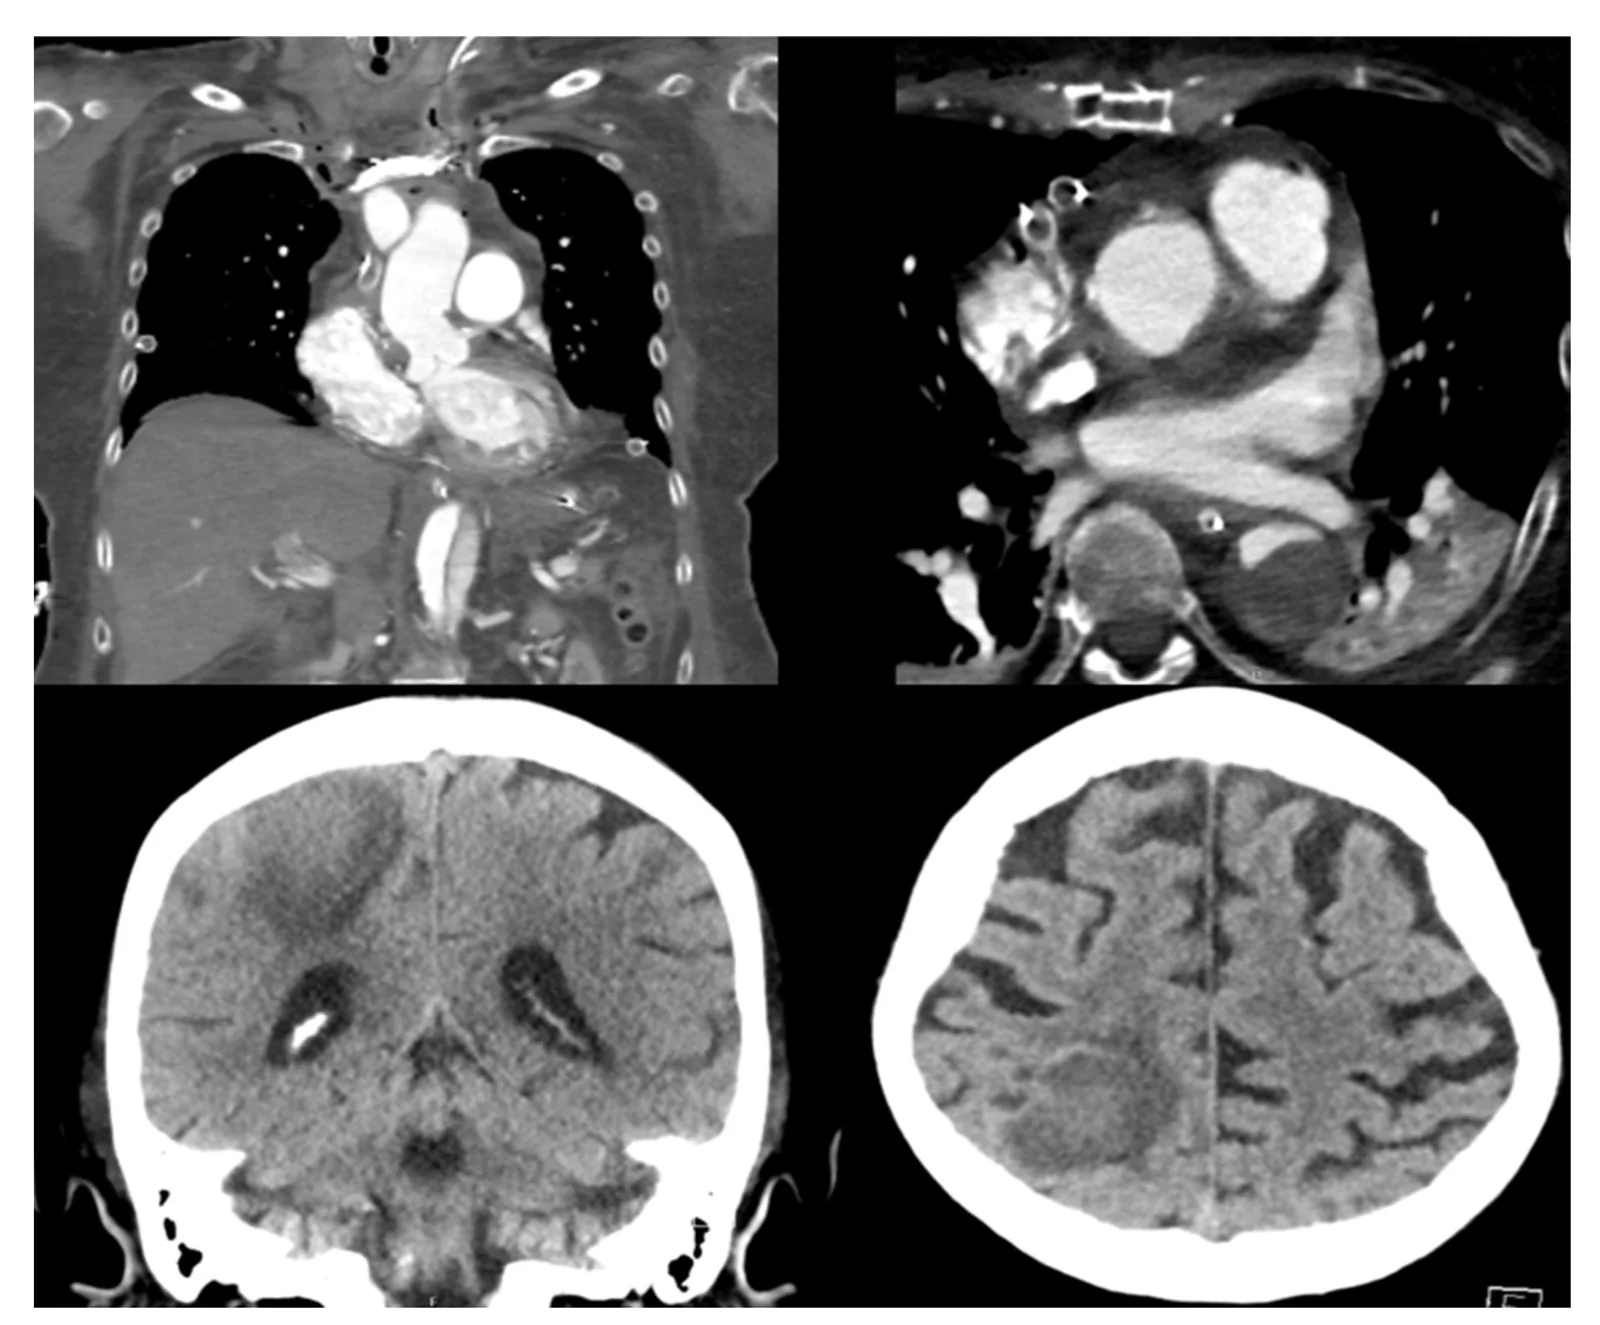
Postoperative computed tomography demonstrating regression of the cerebral haemorrhage and correct positioning of the prosthesis after supracoronary hemiarch replacement of the ascending aorta.
